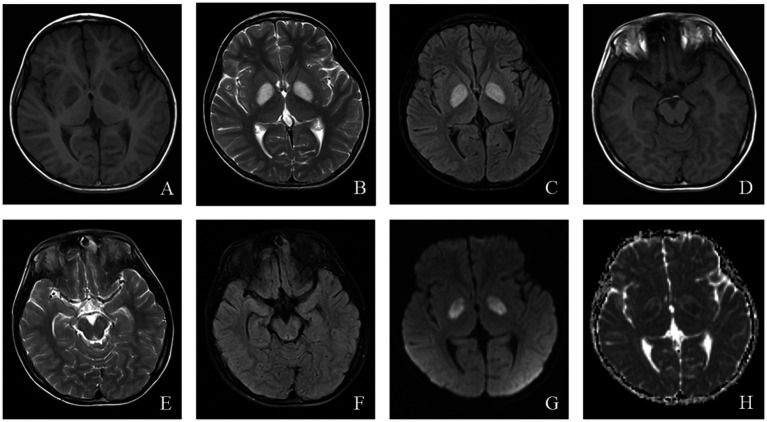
An 8-year-old male who experienced intermittent fever and limb weakness for over 6 years and drowsiness for 1 day was diagnosed with Leigh syndrome. Head MRI showed the following: (A–F) On axial T1WI, patchy low signal intensity was seen in the bilateral globus pallidus and midbrain, while patchy high signal intensity was observed in the bilateral globus pallidus and midbrain on axial T2WI and T2/FLAIR. (G) High signal intensity in the focal area was observed on DWI. (H) ADC showed high and low signal intensity in the corresponding lesions.
